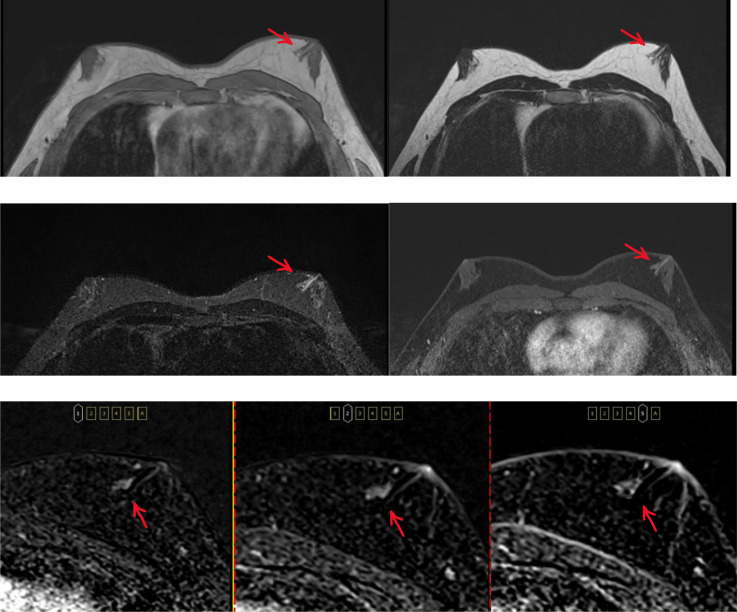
Axial MR images show a dilated duct with internal hemorrhagic products signal-intensity in the subareolar region of the left breast (arrow). First row: pre-contrast T1WI (left), T2WI (right). Second row: STIR (left), T1WI fat suppressed (right) In the left periareolar region there is an area of focal heterogeneous non-mass enhancement in the inner vicinity of the dilated duct (Third row: axial subtraction from left to right: phases 1st, 2nd, and 5th).
